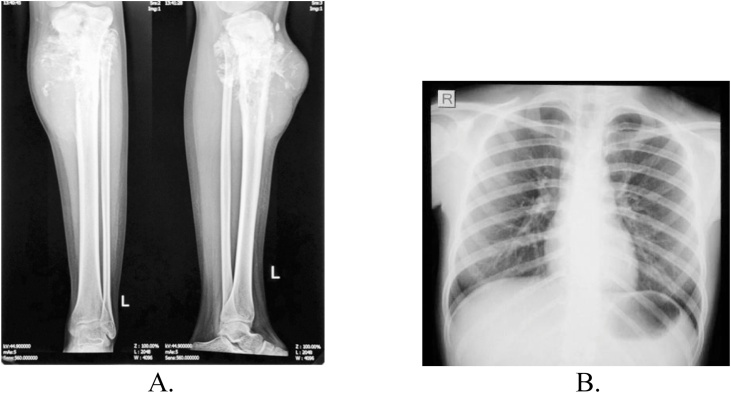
A. Cruris xray showed a mixed lytic and blastic lesion. B. Thorax xray showed no sign of metastasis.
